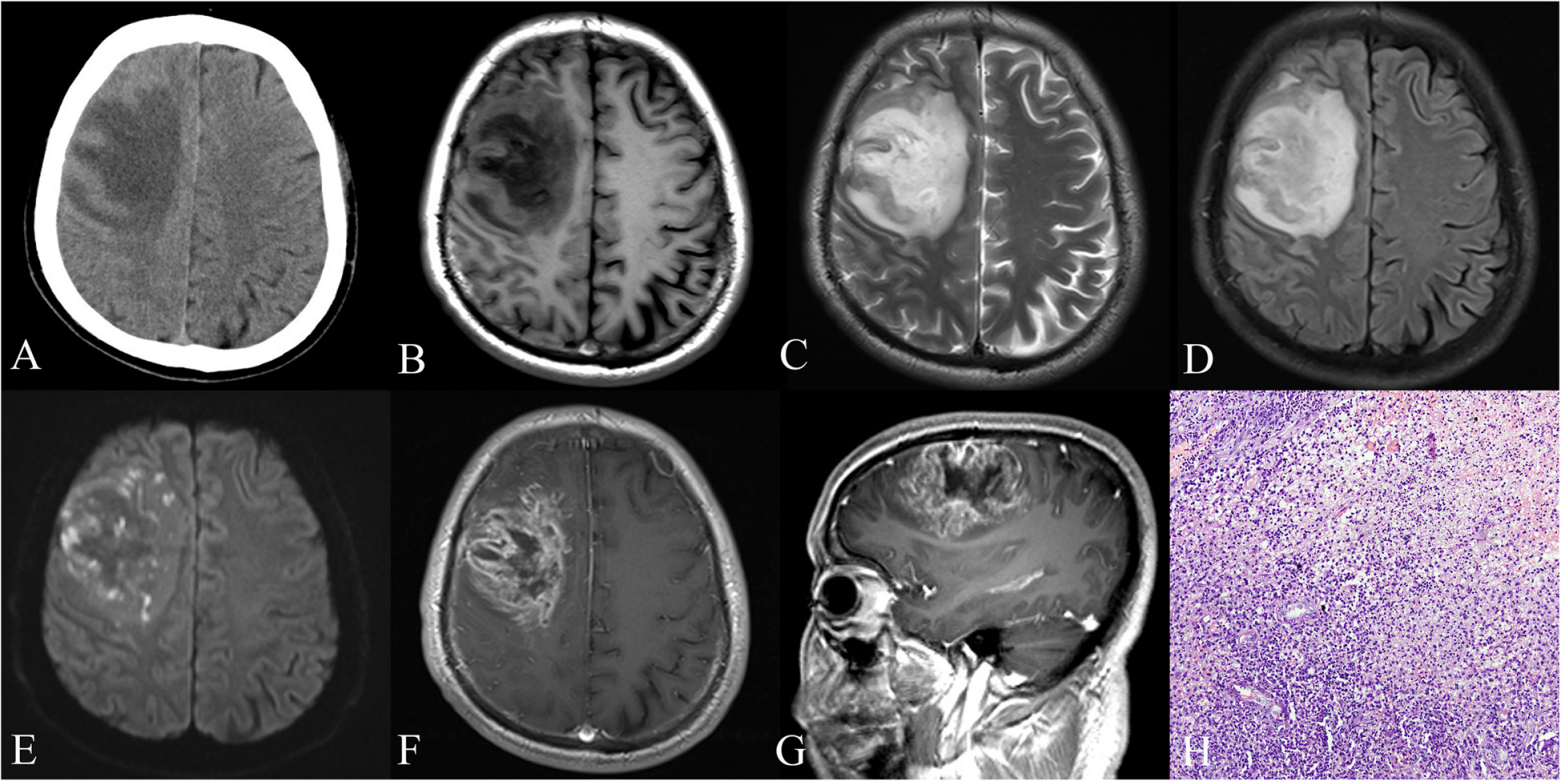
Imaging features of the case: Unenhanced CT (
